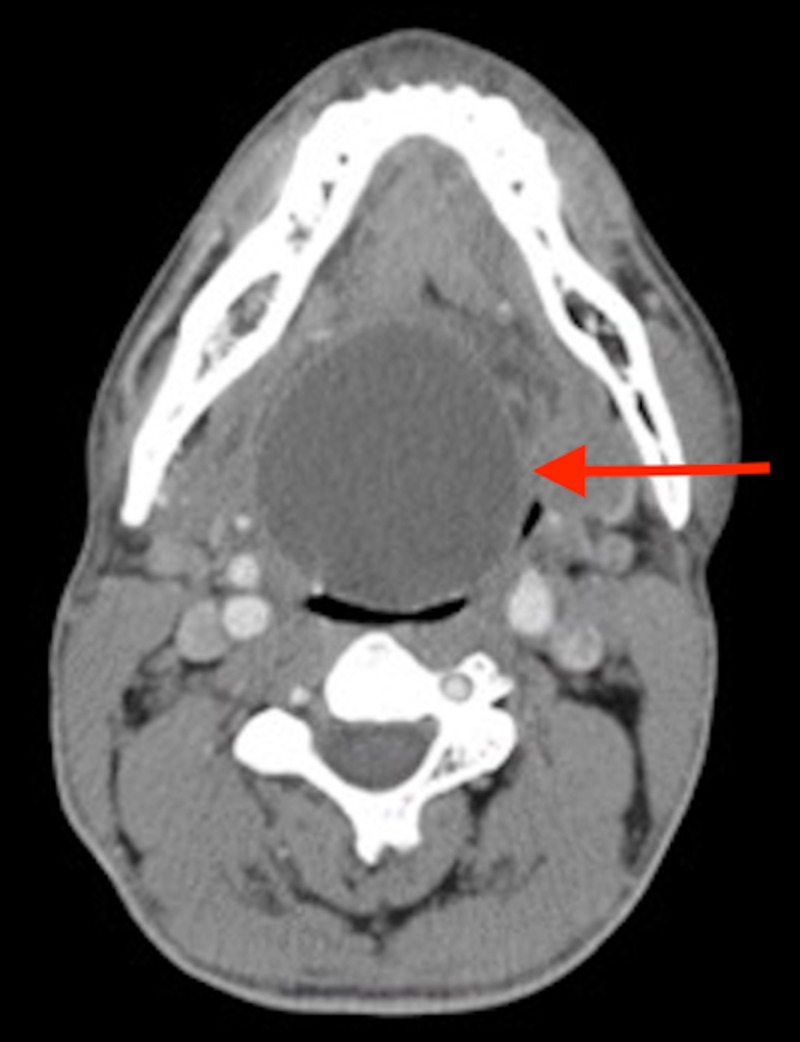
CT image of the mass lodged in the oropharynx, as indicated by the red arrow.
